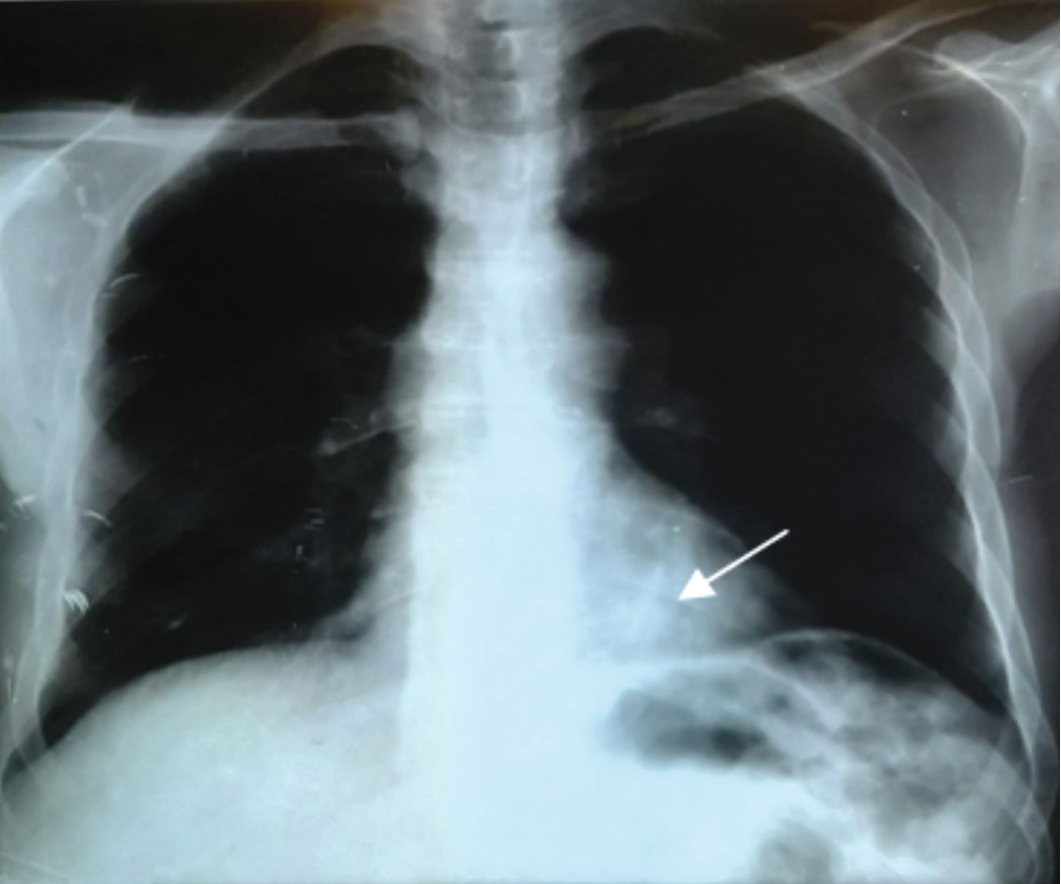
Chest X-ray face (solitary pulmonary nodule of the heart-phrenic angle).
